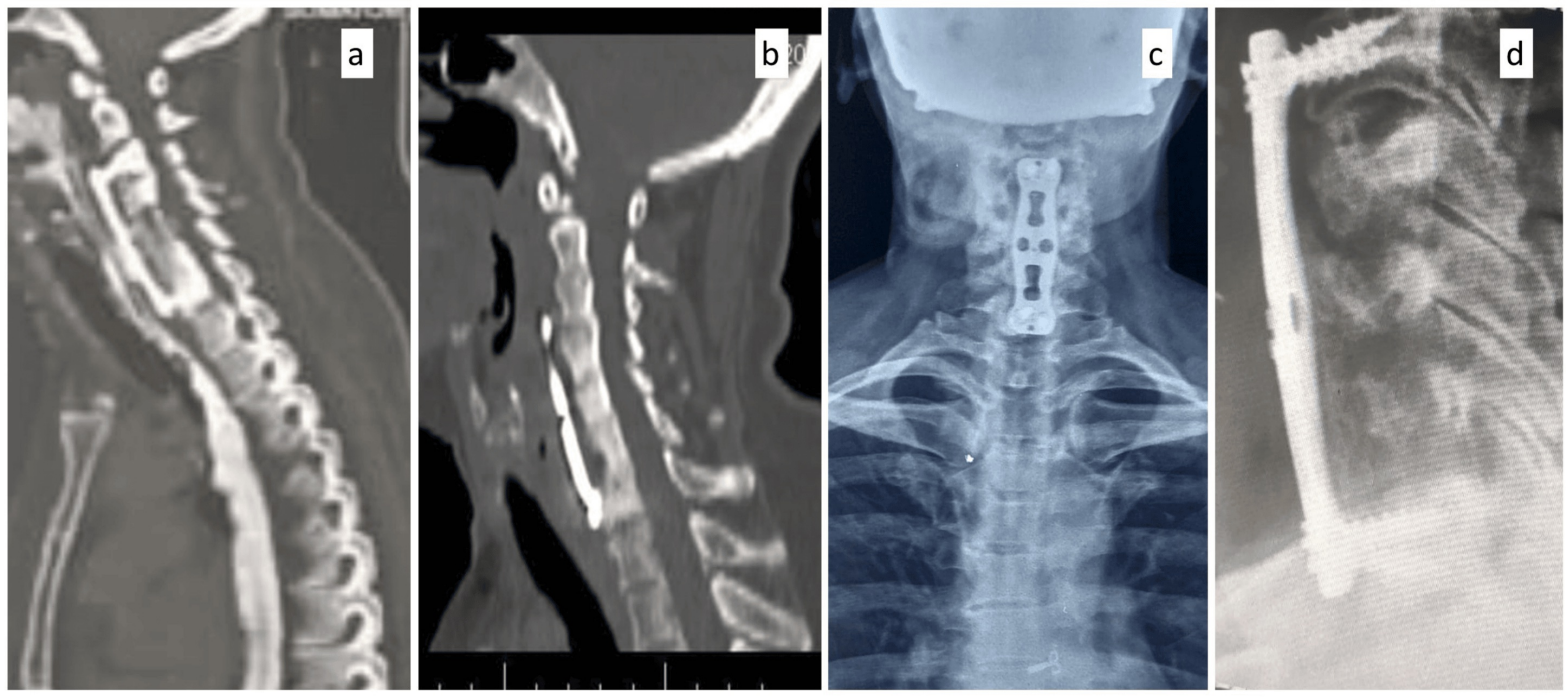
Postoperative computer tomography scans and radiographs after revision surgery showing extension of the fusion from C3 to C7 six weeks postoperatively. The patient was re-evaluated six weeks after the second surgery with a computer tomography scan (CT scan) and radiographs; (a-b) sagittal CT image, (c) anteroposterior radiograph, and (d) lateral radiograph at six weeks postoperatively.
